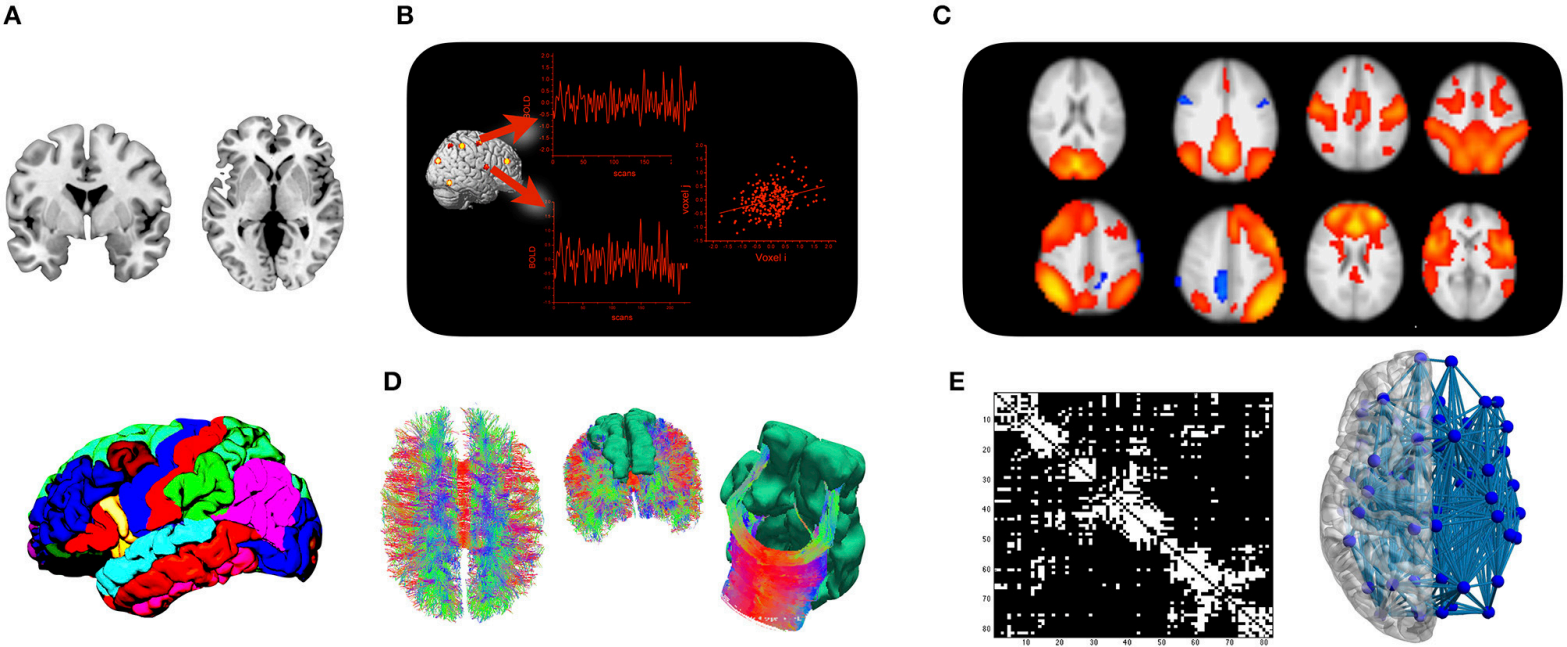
The connectome approach: (A) Cortical and subcortical gray matter are parcellated into a set of regions of interest. (B) Mean time courses of BOLD activity are extracted from each region of interest, and functional connectivity is assessed by analyzing statistical dependencies between any two regions. (C) Functional connectivity is organized into a set of large-scale networks at the brain level. (D) Fiber tracking is applied to diffusion MRI data to assess whether regions from the parcellation are structurally connected. (E) Results are either displayed in a connectivity matrix whose elements indicate whether two regions are connected or not, or displayed in a connectivity plot for anatomical reference.
